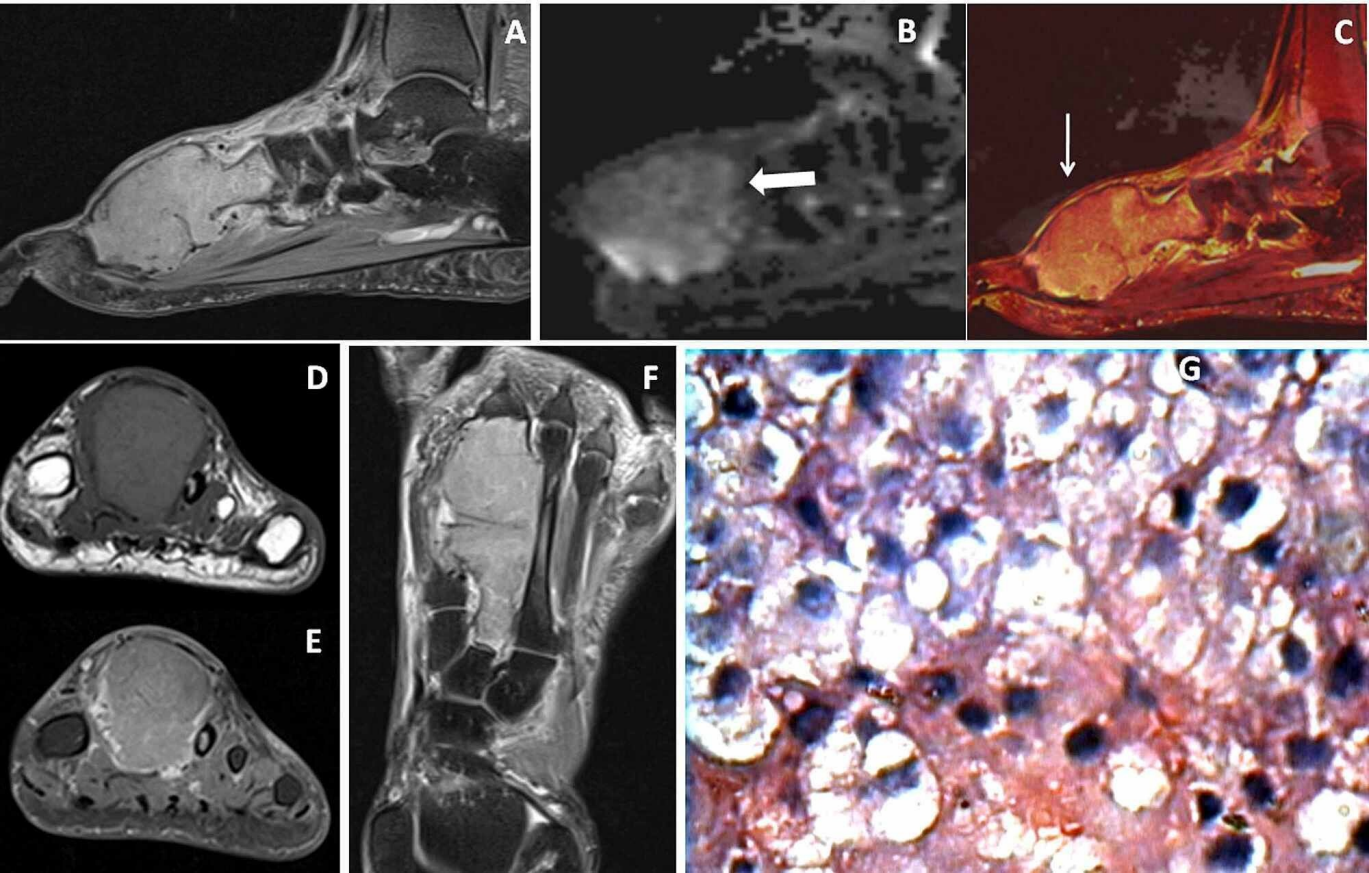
Acral metastasis Sixty-six-year-old male had swelling in his left foot. Sagittal proton density fat-suppressed (PDFS) image (A) showed a hyperintense expansile mass lesion in the second metatarsal bone extending into articular surfaces. Sagittal diffusion-weighted image (DWI) (B) showed diffusion restriction (←block arrow) with bright signals on fusion image (C) between sagittal PDFS and DWI (↓ arrow). Coronal T1 weighted (T1W) image (D) showed expansion of the affected secondary metatarsal with pressure erosion over the third metatarsal bone. Coronal and axial post gadolinium fat-suppressed T1W images (E&F) showed moderate homogenous enhancement of the mass. 40X HPE (image G) showed metastasis.
